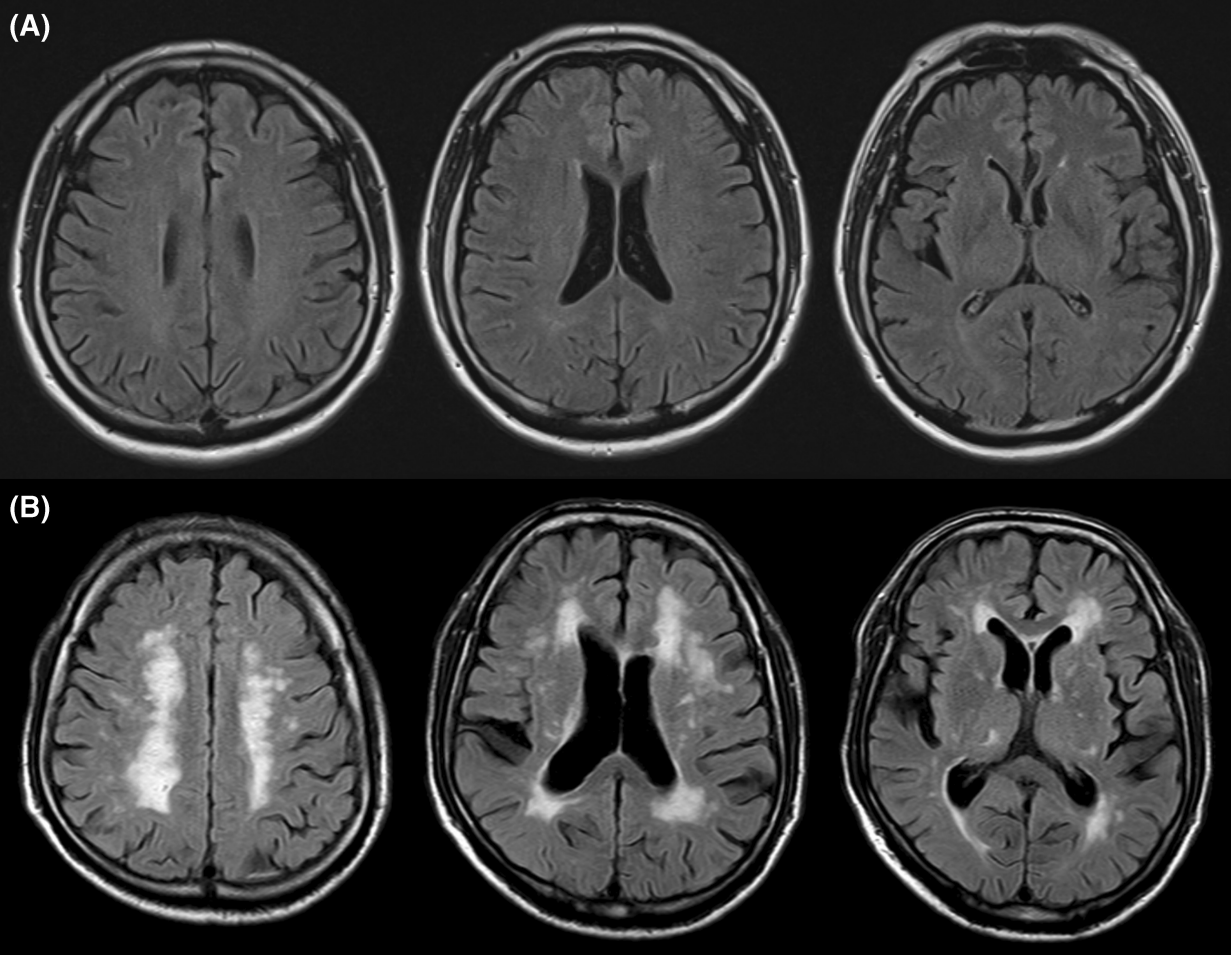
Representative cases. Panel A demonstrates the brain MRI of a 68‐year‐old man with CHIP with *DNMT3A* mutation (variant allele fraction VAF value of 5.8%) and well‐controlled hyperlipidemia (total cholesterol level of 182 mg/dL and LDL cholesterol level of 87 mg/dL) but without other cerebrovascular risk factors. The normalized value of total white matter hyperintensity (WMH) volume was 0.2%. Panel B demonstrates the brain MRI of a 66‐year‐old man with well‐controlled diabetes (hemoglobin A1c of 5.4%) but without CHIP or other cerebrovascular risk factors. The normalized value of total white matter hyperintensity (WMH) volume was 5.9%.
